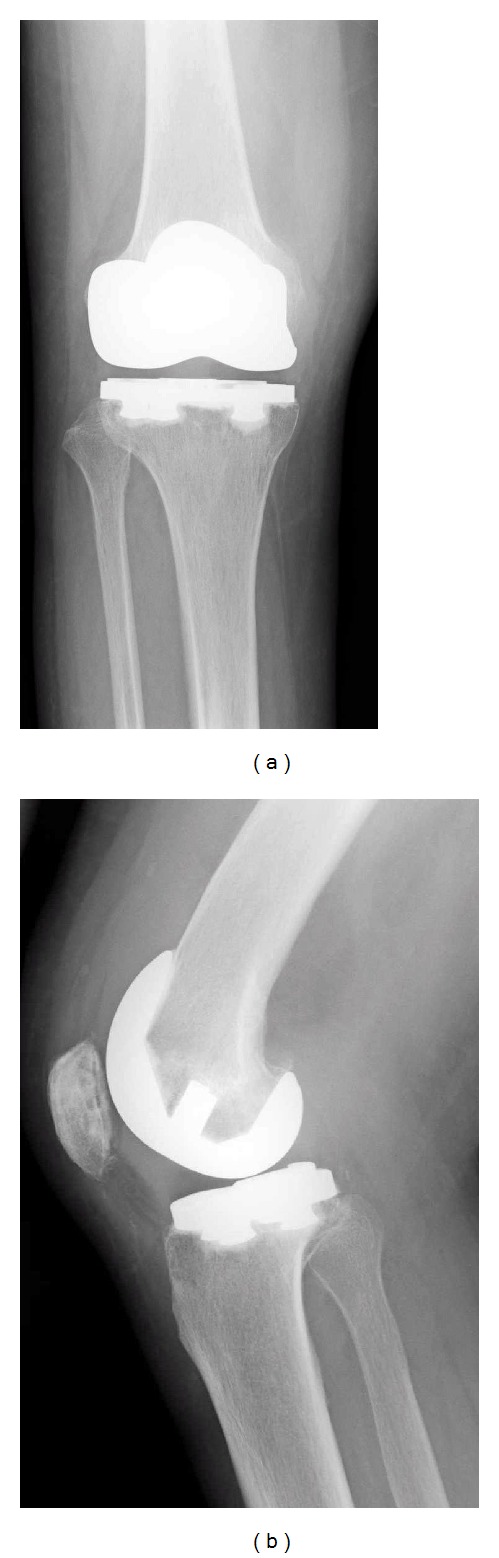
Anteroposterior (a) and lateral (b) radiographs of the patient's right knee at the first visit. No signs of infectious loosening or osteolysis around the prosthesis were detected.
